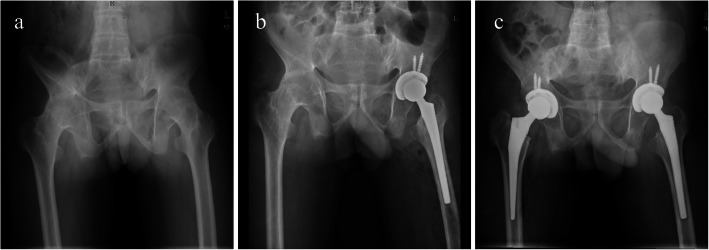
Case presentation of sequential cementless bilateral total hip arthroplasty for osseous ankylosed hips with ankylosing spondylitis. a: A man was diagnosed with ankylosing spondylitis and showed bilateral osseous ankylosed hips preoperatively. b: The radiograph of the pelvis before contralateral THA showed excellent prosthesis position and size of firstly performed THA. c: The film of the pelvis at 97-month follow-up showed superior radiological parameter and good fixation of the prosthesis
